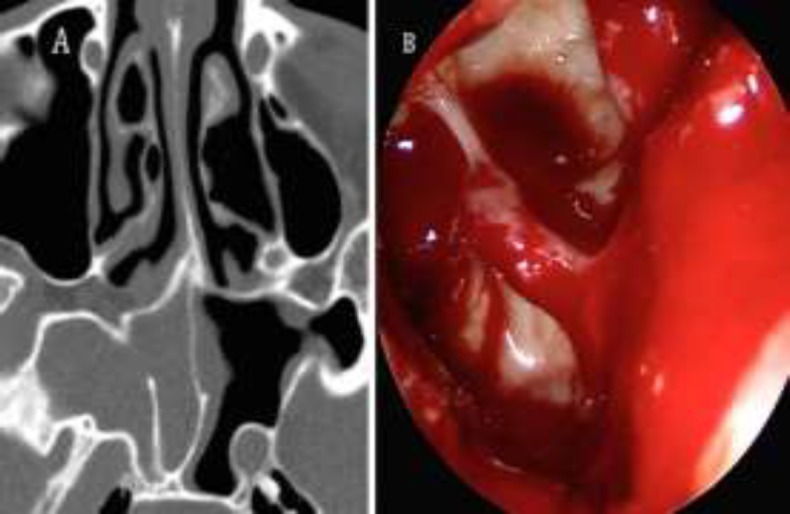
(A) Axial CT scan displays a complete opacification of the right sphenoid sinus, (B) hyperpneumatization of the pterygoid recess, and corresponding endoscopic endonasal surgery image
